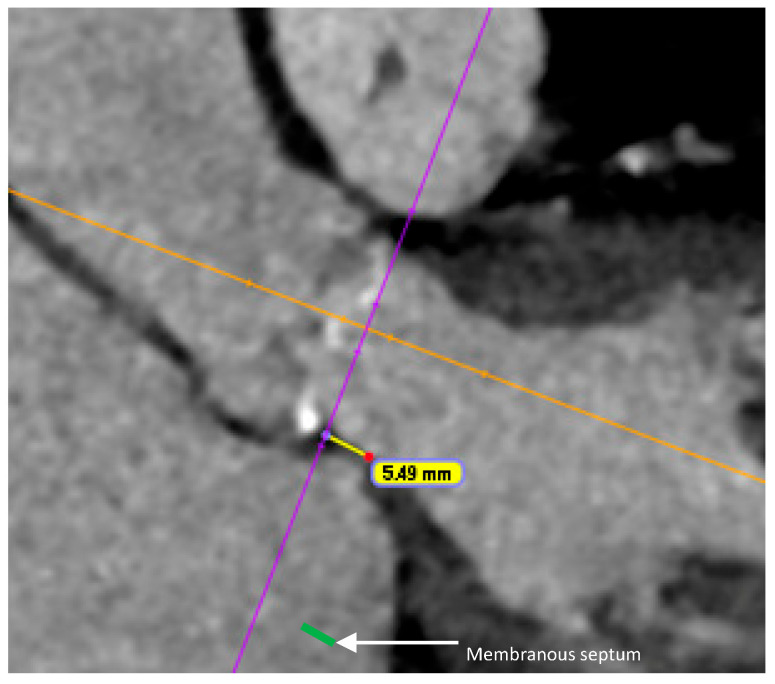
Length of membranous septum (5.49 mm) measured on coronal view of CT scan.
